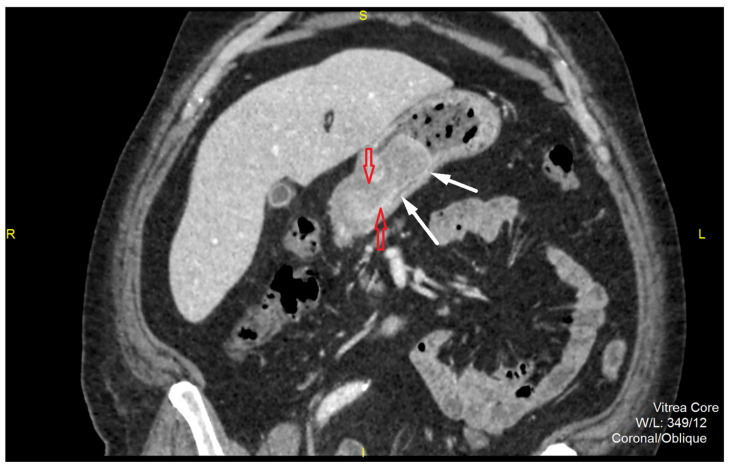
Contrast-enhanced CT showing a well-circumscribed enhancing intraluminal prepyloric gastric mass (white arrows) measuring 35 × 40 × 60 mm. The lesion demonstrates homogeneous enhancement without necrosis, cystic degeneration, or calcification, and the overlying mucosa appears preserved. Limited gastric distension due to patient intolerance hindered complete evaluation of the lesion attachment (red arrows).
